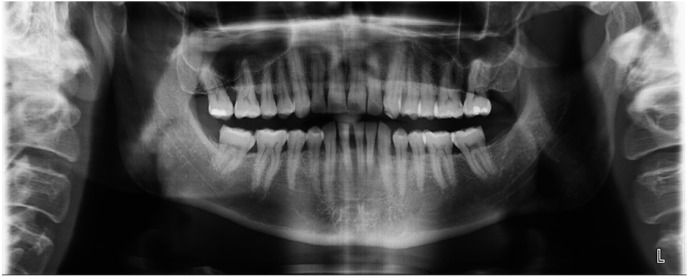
Orthopantomography made on the first visit. No abnormalities that may explain the neuropathic symptoms were observed.
